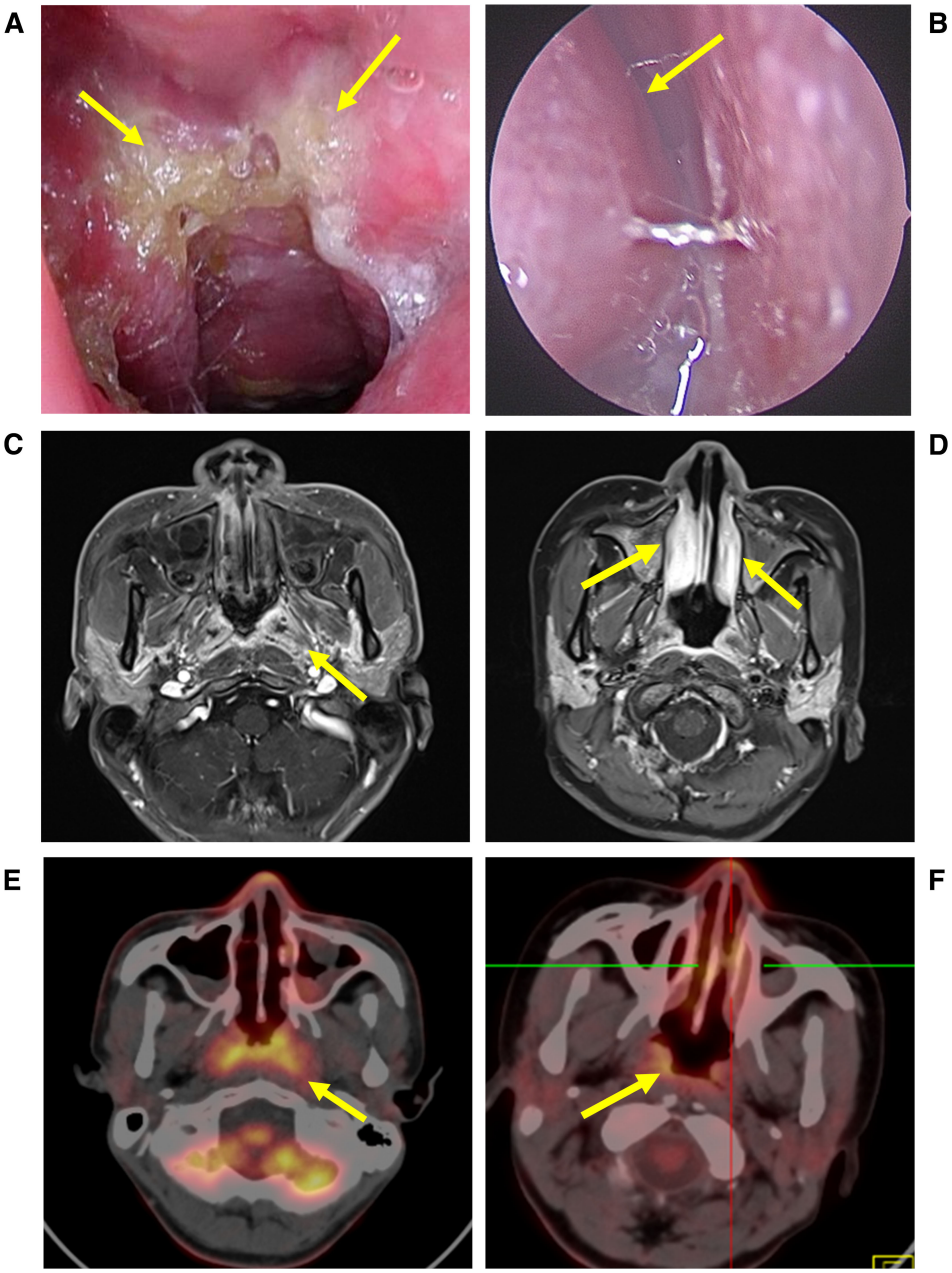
Nasal endoscopy and imaging findings of the two patients. (A) Nasal endoscopy of the patient revealed lymphoid hyperplasia in the nasopharynx, the pharyngeal recess and the torus tubails were indistinct. (B) Inferior turbinate swelling with retention of secretions. (C) Enhanced MRI: 30% thickening of soft tissue on the posterior wall of the nasopharynx, mild to moderate enhancement after intravenous injection of contrast agent. Shallow bilateral pharyngeal recess. Inflammatory effusion in bilateral maxillary sinuses. (D) 40% thickening of the right inferior nasal turbinates, inflammatory effusion of bilateral maxillary sinuses. No significant thickening of soft tissues in the nasopharynx, and no significant enhancement in the nasal cavity enhanced scan. (E) The mucosal thickening of the lateral and posterior walls of the nasal cavity exhibited a concentrated distribution of radioactivity (SUVmax approximately 10.8). (F) Slightly thickened radioactivity distribution in the nasopharynx (SUVmax about 8.3). A small soft tissue shadow was seen in both nasal cavities with relatively concentrated radioactivity distribution (SUVmax about 6.3).
